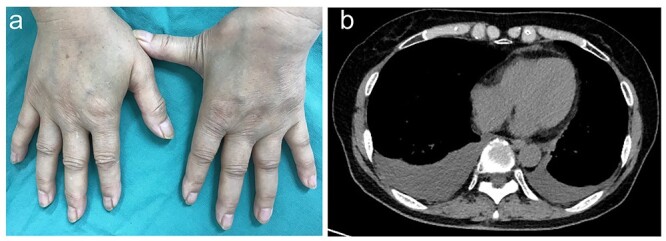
key clinical images. (a) Dorsal view of diffusely swollen hands with pitting edema. (b) Chest CT scan showing bilateral pleural effusions.
